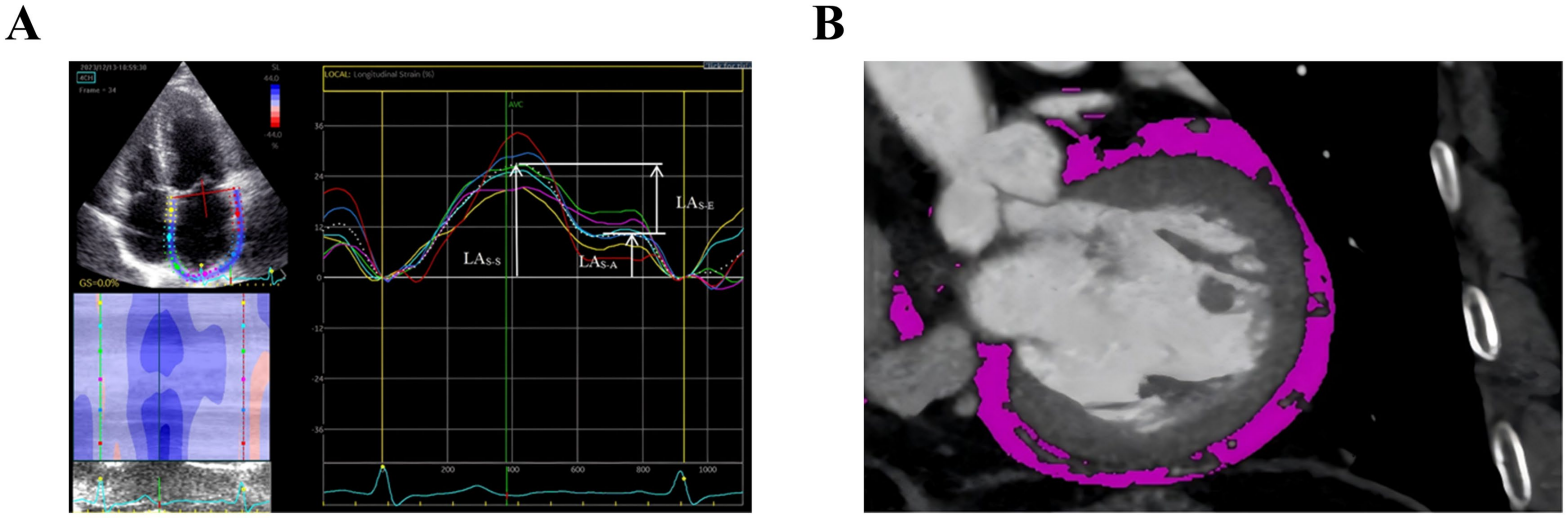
(A) Two-dimensional speckle tracking echocardiography (2D-STE) derived LA strain analyses from the apical four-chamber view. (B) EATV quantification assessed by coronary computed tomography angiography (CCTA).
